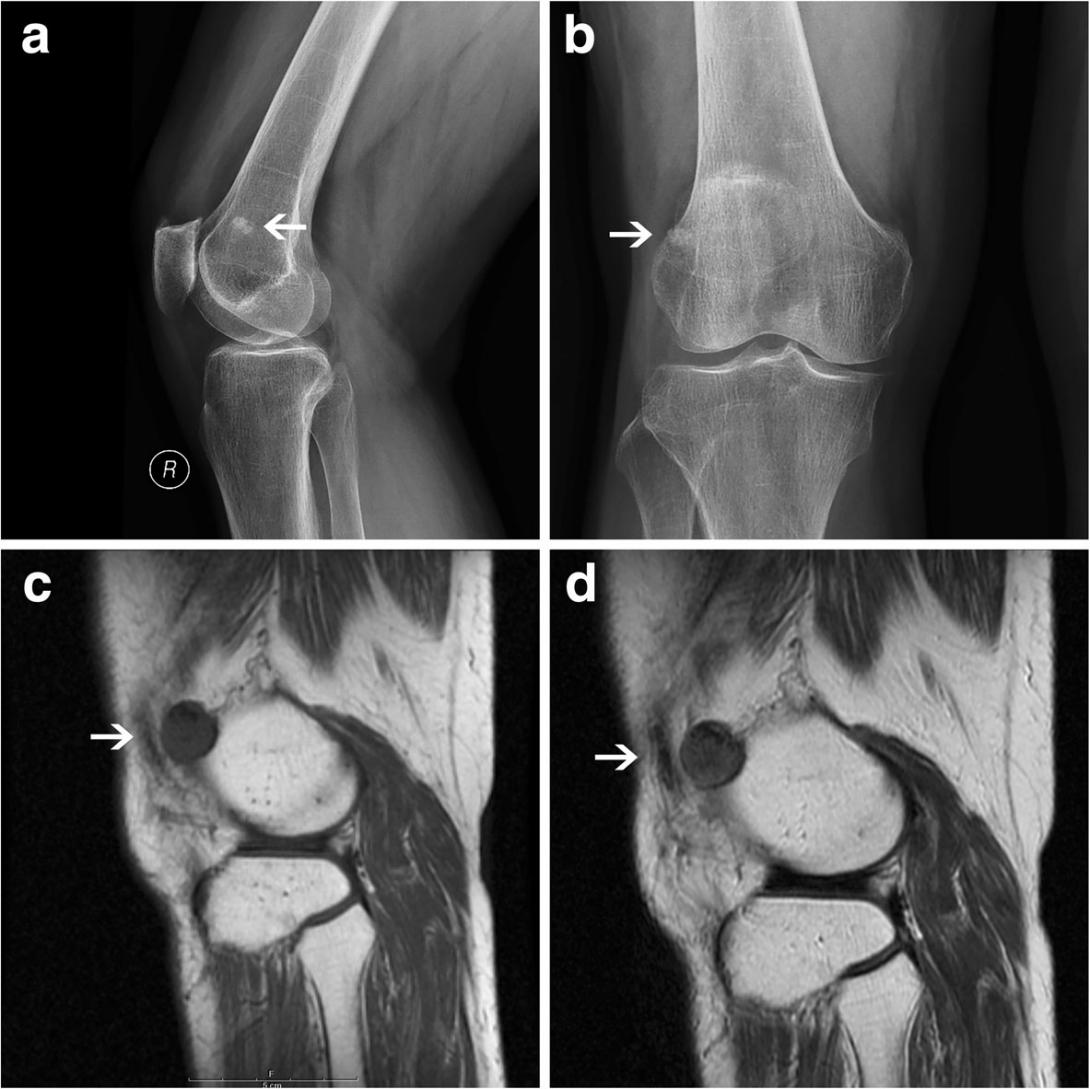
Radiograph plain film showed a “loose body” beside the lateral femoral condyle in the right knee (a, b). MRI showed the signal of the nodule was not uniform and isointense to muscle on T1WI (c) and T2WI (d)
